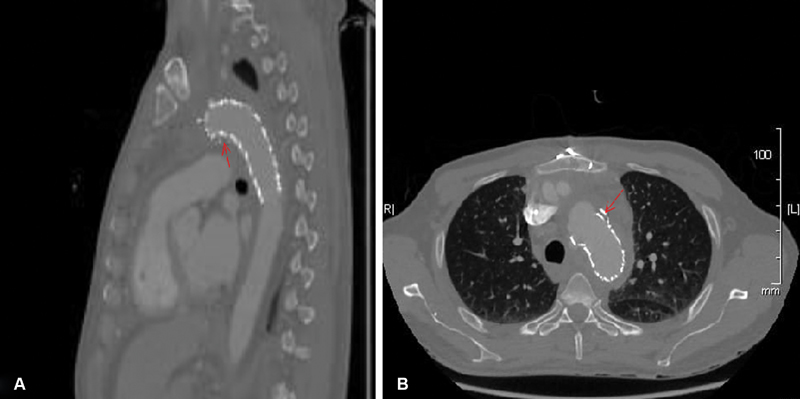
Computed tomography for chest showed postcollapsed stent graft endovascular treatment by reinsertion for other stent graft and ballooning as showed in the arrow in sagital view (
